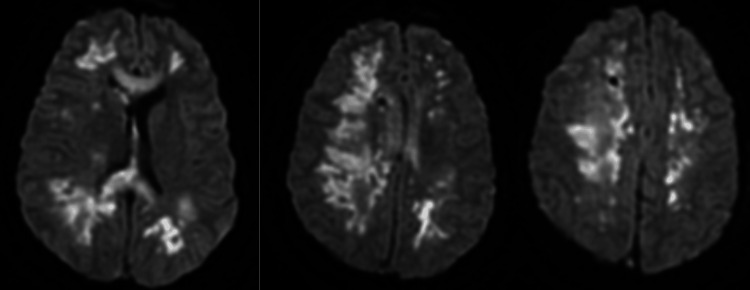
Brain MRI Imaging demonstrating multiple watershed supratentorial and basal ganglia infarcts and left temporal lobe hemorrhage.
